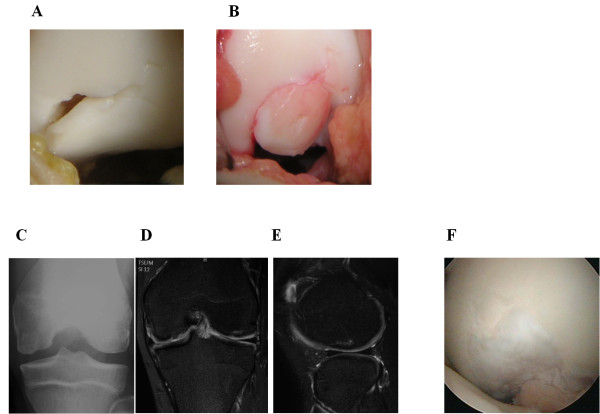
Operative and postoperative findings in case 2. (A) Arthroscopy shows that the free margin of a chondral fragment still partially attached to the femoral condyle posteriorly. The anterior margin of the femoral condylar defect is seen. (B) An osteochondral plug is grafted into the recipient site. (C) Anteroposterior radiograph taken two year after surgery shows less irregularity of the subchondral bone of the medial femoral condyle. (D, E) MRI taken two years after surgery shows the restoration of the articular cartilage surface and good engraftment of the graft. (F) Second look arthroscopic finding of the transplanted site demonstrates that the lesion is covered with cartilageous tissue.
